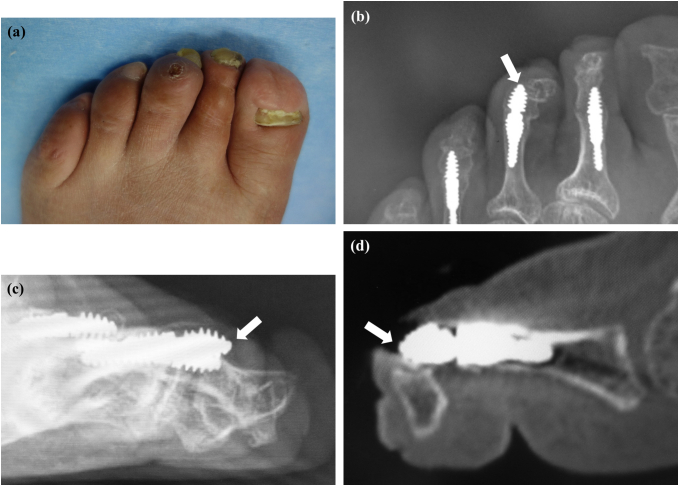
Clinical photograph showing swelling and ulceration over the dorsal surface of the distal interphalangeal joint in the third toe (a). Arrow showing the dorsal edge of the distal portion of the implant penetrating the dorsum of the middle phalanx head in a radiographic oblique view (b) and in lateral (c) and sagittal computed tomography scans (d).
